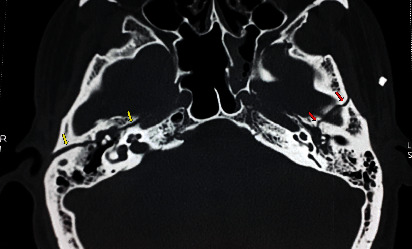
Transverse section of cranial CT (plane of incudomalleolar joint) showing right longitudinal otic-sparing temporal bone fracture (yellow arrows) and left transverse temporal bone fracture (red arrows).
